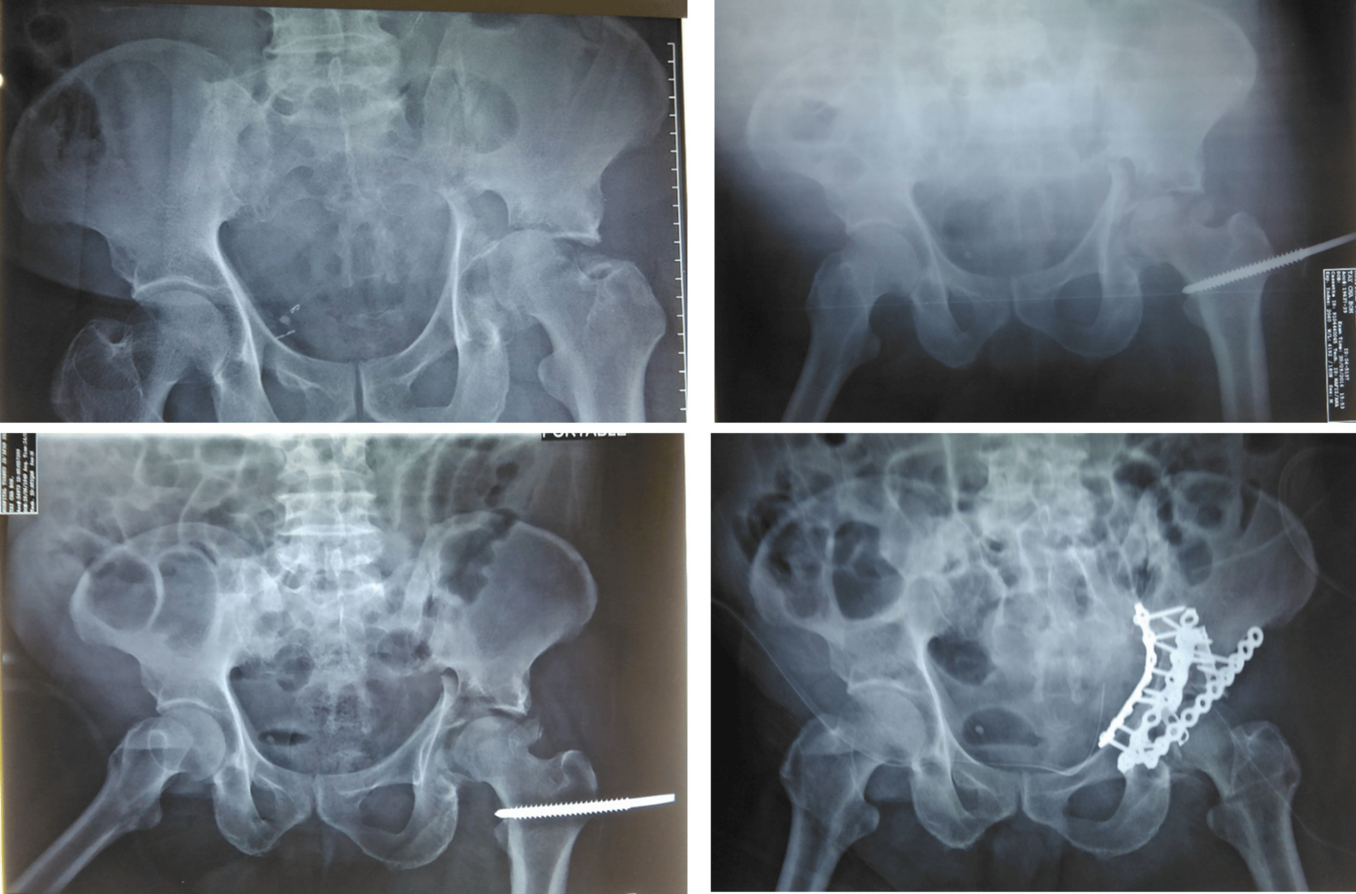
Serial radiograph of the patient before traction application with initial displacement, reduction with traction increment, and post-surgery fracture fixation
